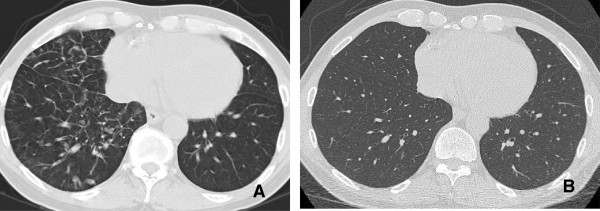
Imatinib induced pulmonary edema. A. CT scan on the chest demonstartes thickening of the interlobular septa in the right lung base with no evidence of pleural effusion. B. Restaging CT scan obtained 1 month later shows resolution of the pulmonary edema after dose reduction.
